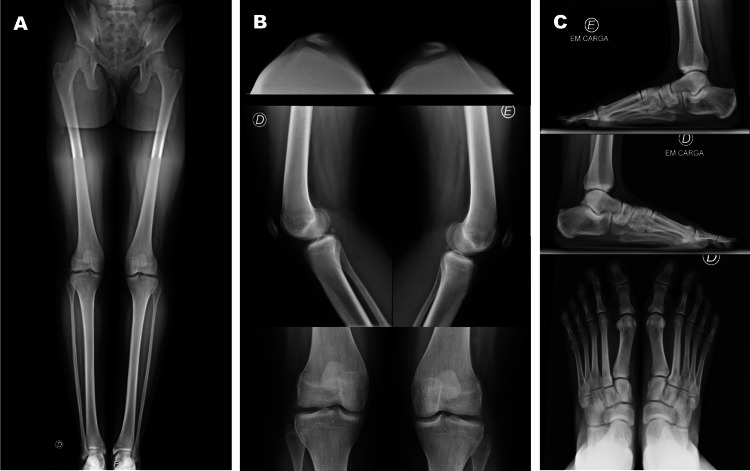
X-rays of the patient: (A) delayed ossification of the ischiopubic junction. (B) bilateral patellar hypoplasia. (C) reduced longitudinal arches of the feet and widened gap between the first and second toes.
